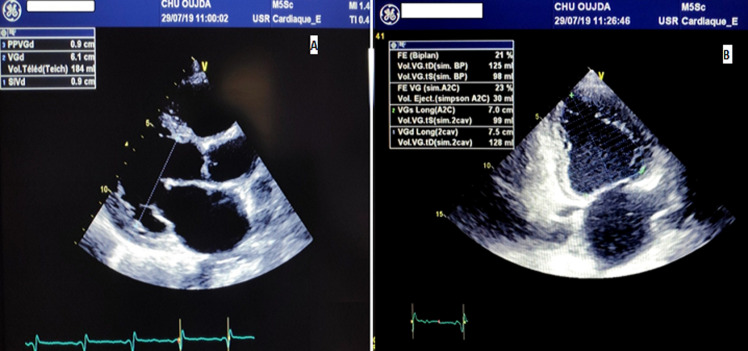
A) transthoracic echocardiography (TTE) in long-axis view showing dilated LF with LVEDD of 61 mm; B) apical 2-chamber, 2-dimensional echocardiogram showing severe systolic dysfunction at 21%
